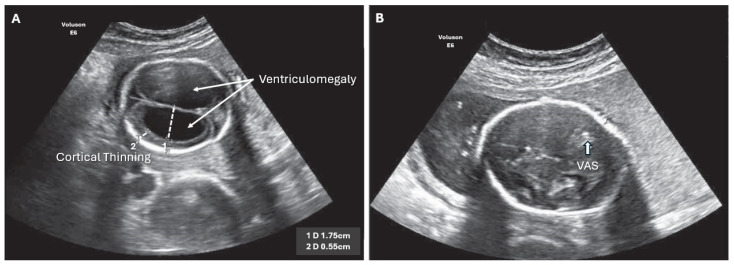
ISVM fetal ultrasound before (A,B) after VAS implantation, arrow indicates VAS.
